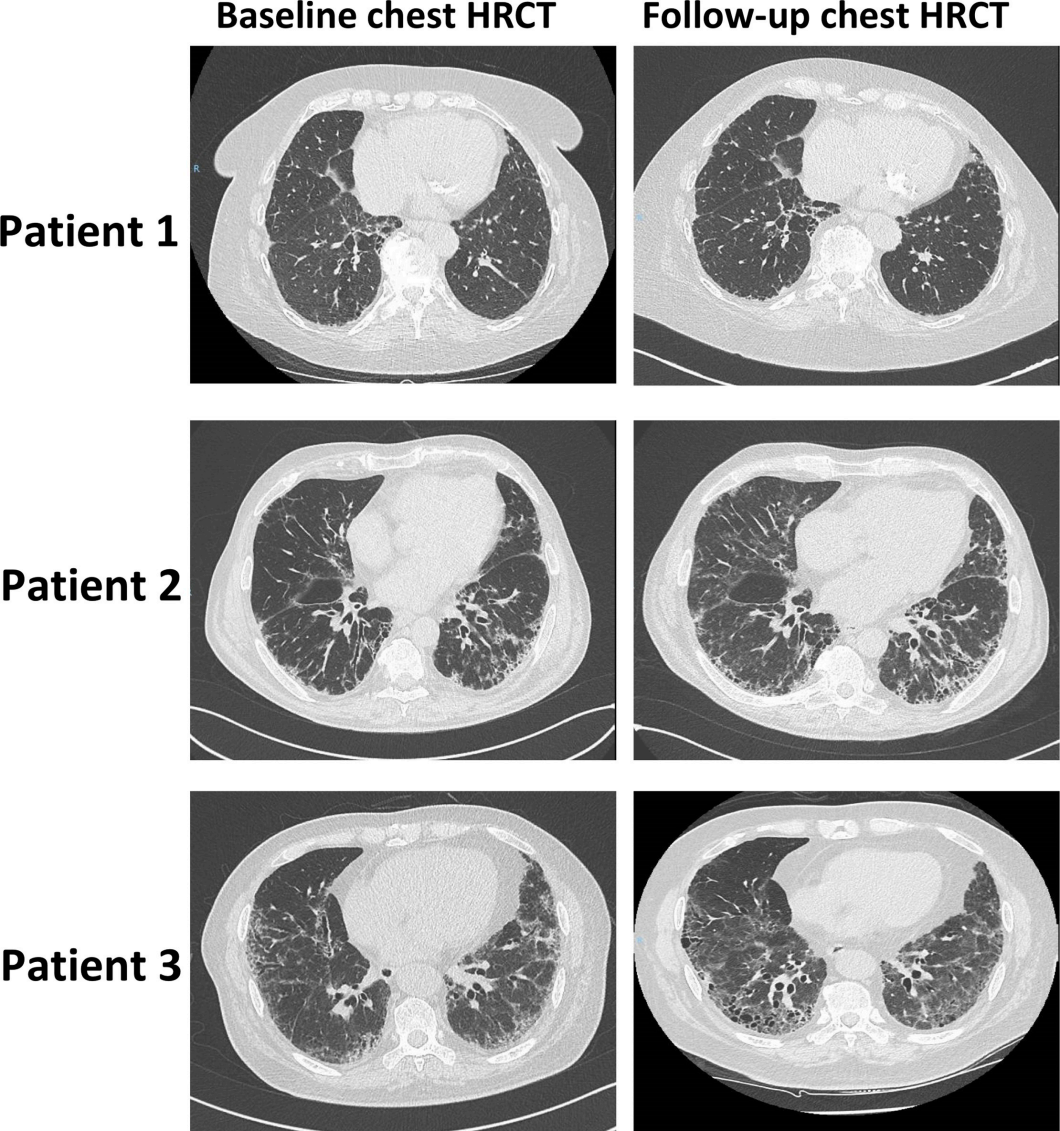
Representative baseline and follow-up chest high-resolution computed tomography performed for 3 patients with rheumatoid arthritis (RA)-associated interstitial lung disease (ILD). Patient 1: baseline KL-6 concentration of 543 U/mL, with no ILD progression detected on chest high-resolution computed tomography (HRCT). Patient 2: baseline KL-6 concentration of 1,412 U/mL, with ILD progression detected on chest HRCT. Patients 3: baseline KL-6 concentration of 4,414 U/mL, with ILD progression detected on chest HRCT.
